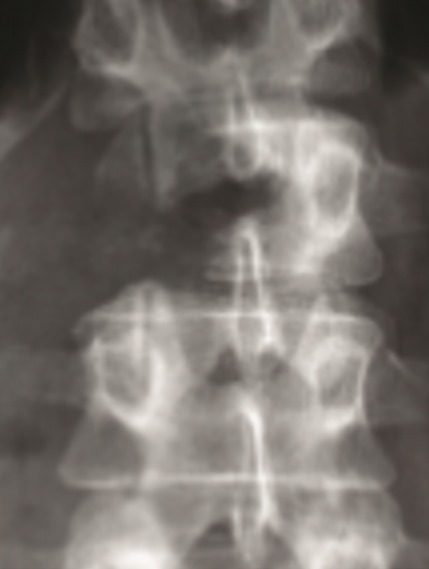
Radiographie standard de face : vertèbre borgne métastatique (le pédicule droit n’existe plus)
